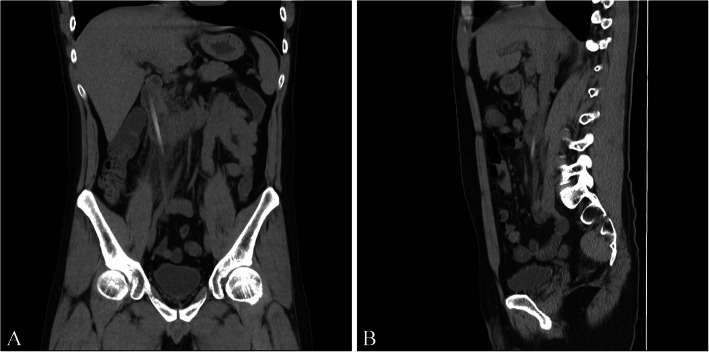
Abdominal computed tomography at the time presentation. A long, radiopaque foreign object was found in the second portion of duodenum piercing into retroperitoneal cavity. a) Coronal view b) Sagittal view
